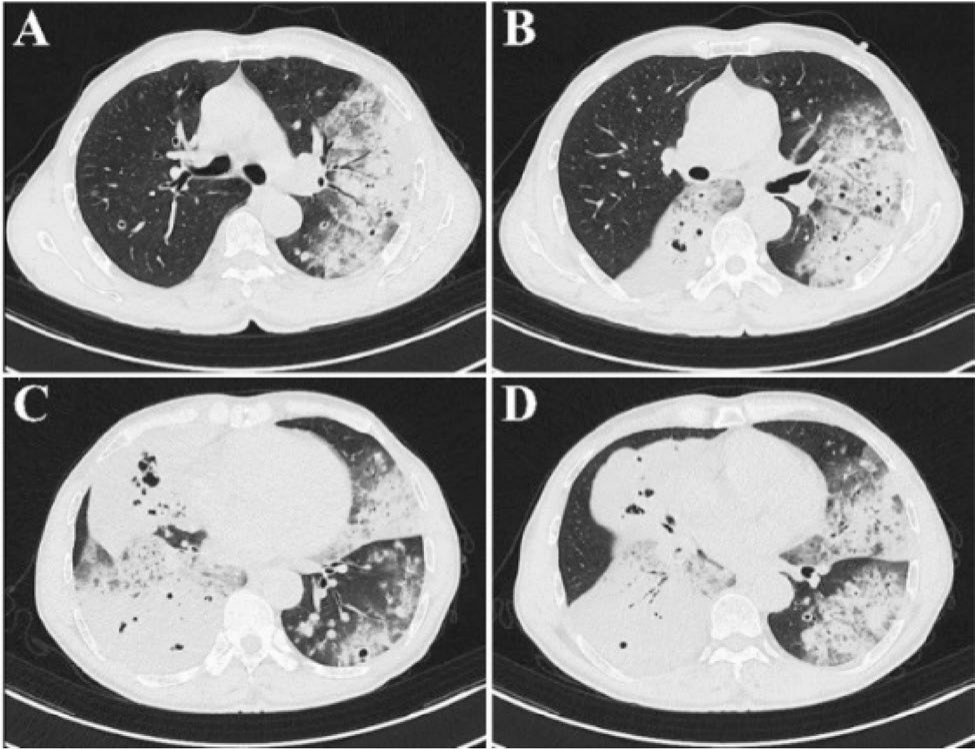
Contrast-enhanced CT images (A-D) during first admission demonstrate bilateral diffuse patchy opacities and consolidation with multiple cavities, predominantly affecting the right middle-lower lobe and left lung, with associated nodules
